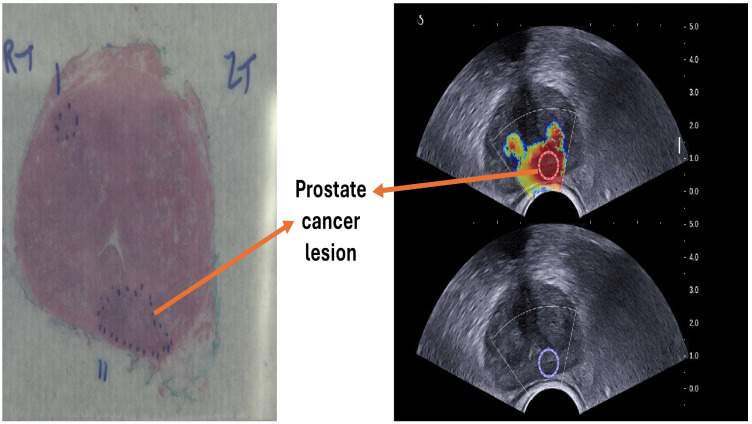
Visualization of prostate cancer lesion in the SWE and B-mode ultrasound (Right side), confirmed by radical prostatectomy image (Left side).
